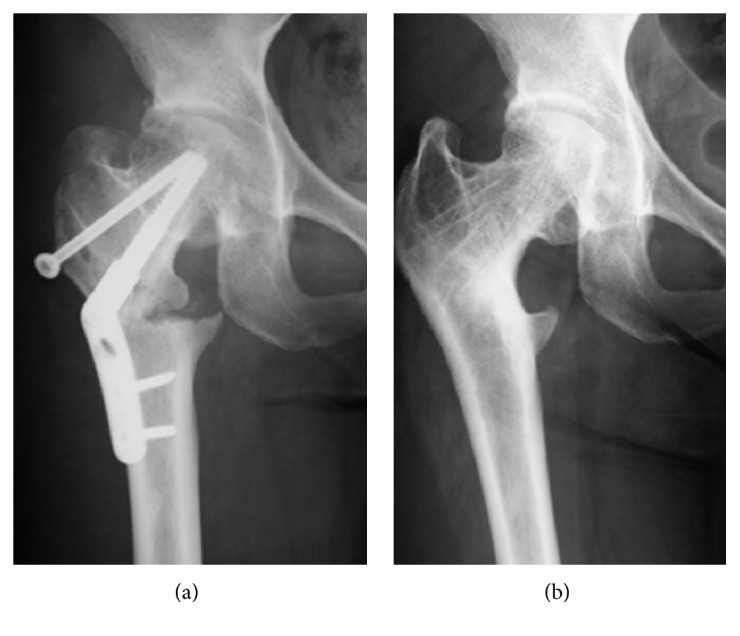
Case 2. (a) Anteroposterior X-ray image of the right hip after femoral curved varus osteotomy, (b) 9 years after osteotomy, X-ray image showing a spherical femoral head with joint space and remodeling of the femoral neck.
